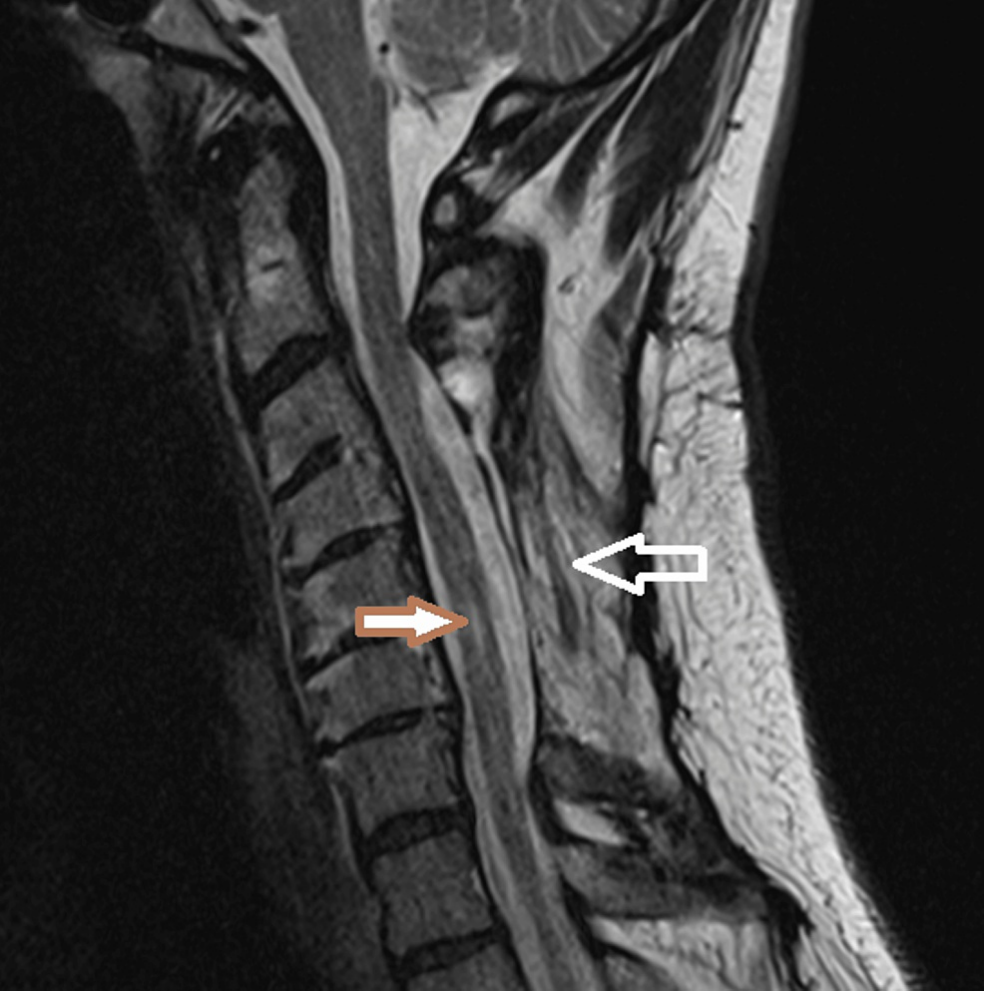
MRI cervical spine, sagittal view T2-weighted image, showing chronic postoperative changes related to previous procedures of posterior spinal fusion and decompressive laminectomies (white arrow) and area of myelomalacia at C5-C6 level (brown arrow) MRI - magnetic resonance imaging
